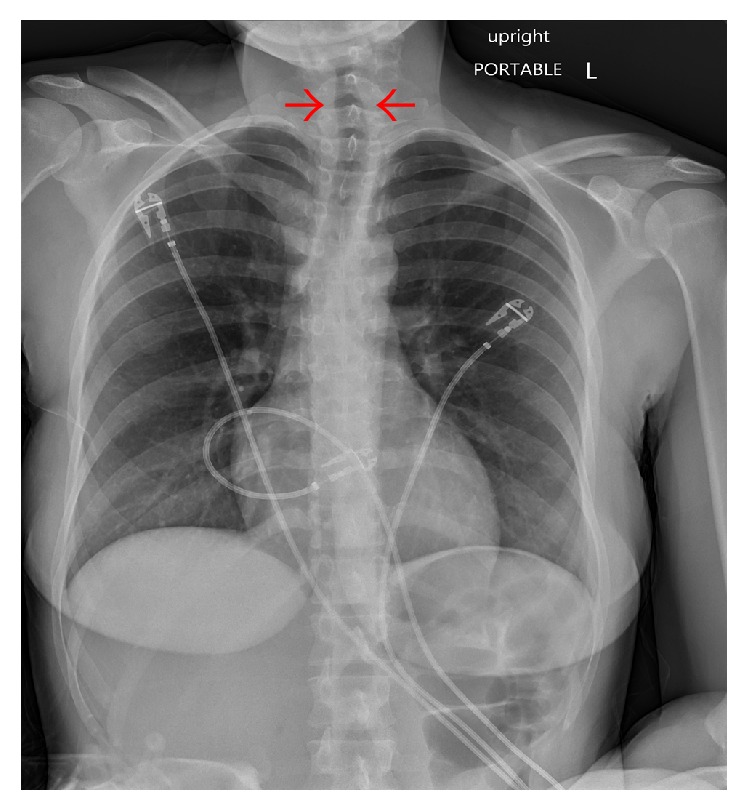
Hospital day 1 anterior-posterior (portable) chest radiograph demonstrating resolution of subglottic stenosis (between red arrows).
